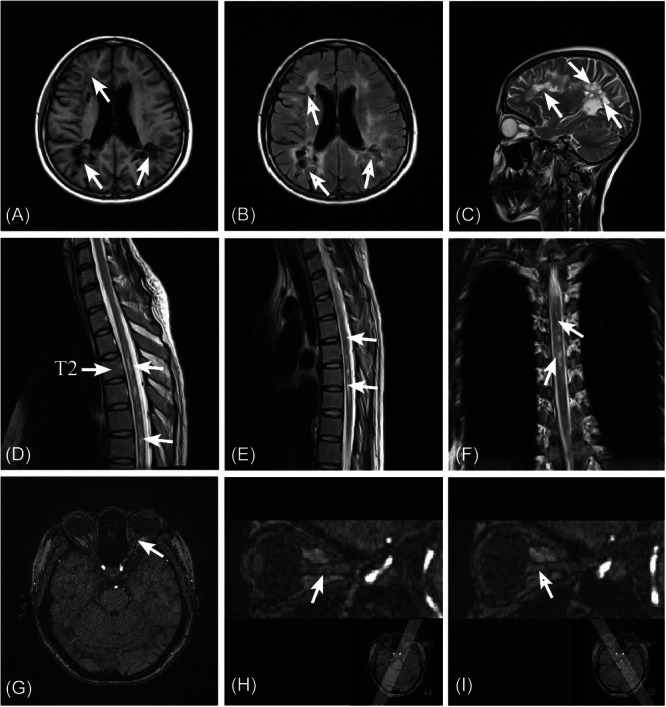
MRI images of brain and thoracic medulla (August 10, 2021, approximately 6 years and 9 months after the initial diagnosis and treatment). (A, C) There were multiple demyelinating plaques with heterogeneous signal in both cerebral hemispheres (white arrow). (B) Some areas in the FLAIR sequence were low‐intensity (white arrow). (D–F) In the upper thoracic segment (approximately flat chest level 2‐6), the spinal cord had a multi‐slice‐length T2 signal, and the contrast‐enhanced scan showed significant enhancement (white arrow). (G, H) The left optic nerve was slightly thickened (white arrow). (I) The right optic nerve showed no abnormality in its course, shape, or signal (white arrow).
